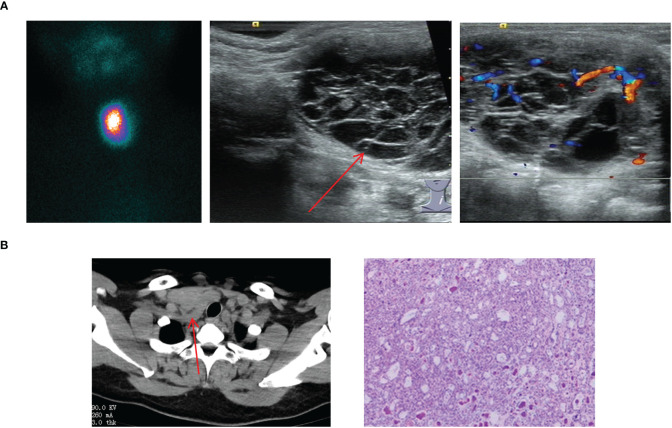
Imaging manifestations and pathological findings of lateral cervical ETG. (A): A 7-year-old girl with a cystic-solid mass in the lymph node of zone IV. The thyroid ultrasound showed a cystic-solid echo with clear boundaries, which had visible blood flow signals; the static thyroid imaging showed abnormal radioactive distribution in the neck. (B): A 51-year-old woman, the postoperative pathology of her right neck mass presented with the adenomatoid nodular goiter. The CT showed a mass with soft tissue density shadow behind the right neck clavicle that had the irregular shape and unclear boundary.
